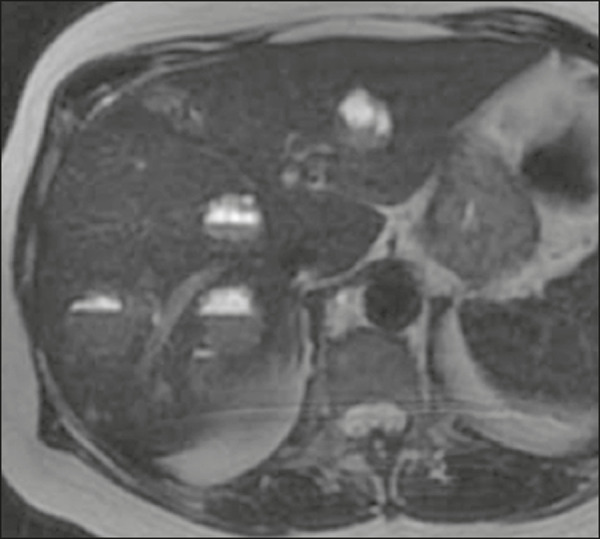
Portal phase CT scan showing a liver metastasis containing foci of calcification.
